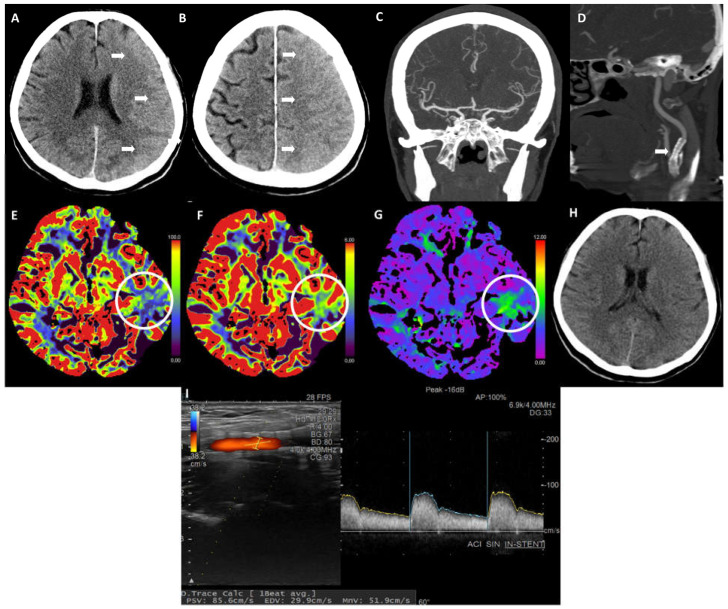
Neurological worsening. (A,B) A post-procedure CT showing sulcal effacement in the left cerebral hemisphere denoting edema (arrows). (C) A CTA post-procedure showing patent intracranial arteries. (D) A CTA post-procedure showing a patent stent in the left ICA (arrow). (E) A CT perfusion (CTP) post-procedure showing attenuated cerebral blood flow (CBF) in the left temporal region (circle). (F) The CTP shows attenuated cerebral blood volume (CBV) in the left temporal region (circle). (G) A CTP showing delayed TMAX in the left temporal region (circle). (H) A follow-up CT two days post-procedure denoting the improvement of the edema in the left hemisphere. (I) A follow-up DUS one month post-procedure showing a patent stent in the left ICA with normal hemodynamics.
